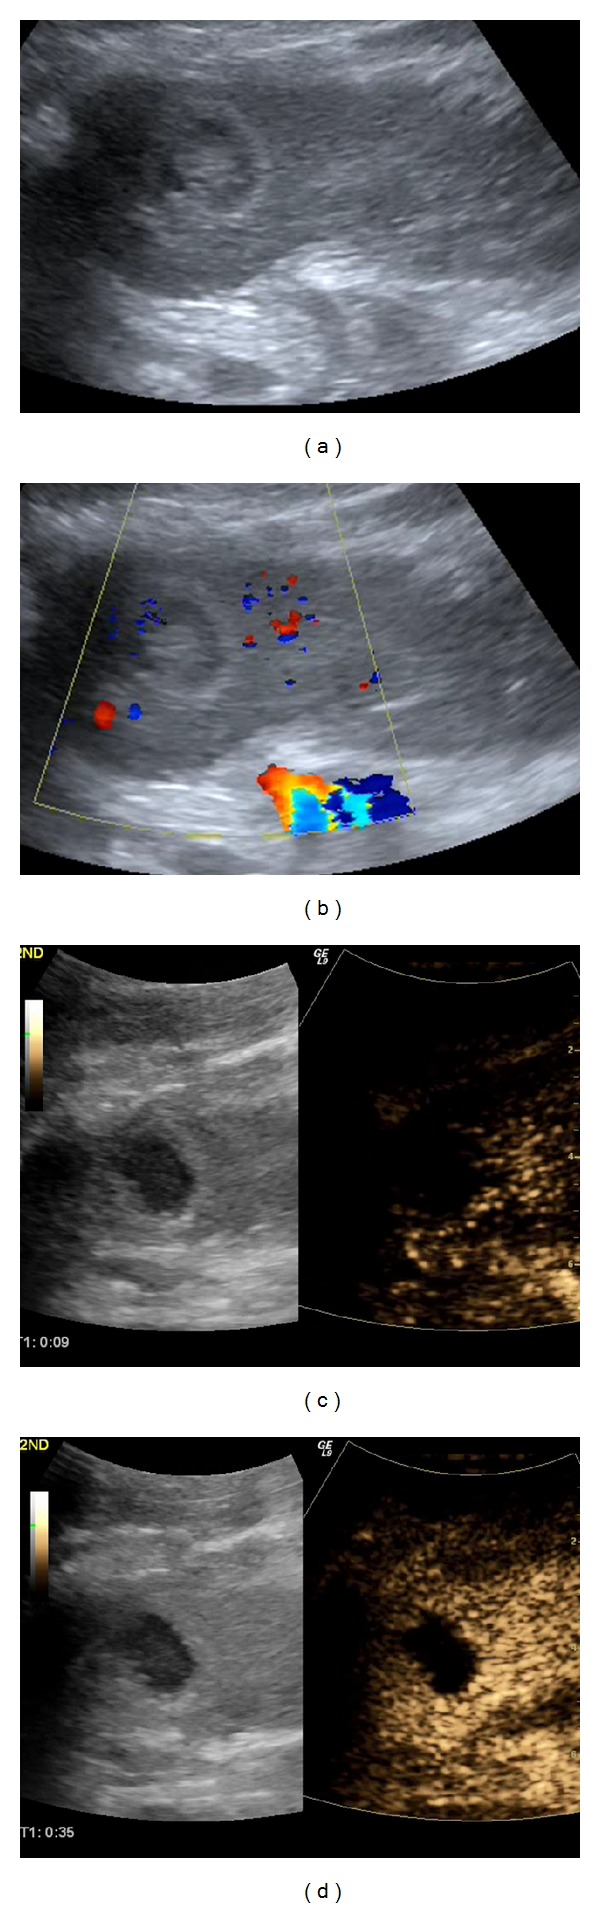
Renal abscess: B-mode (a) and Colour Doppler (b) US detect a round mixed echogenicity lesion in the upper part of the left kidney. After contrast injection, early enhancement is seen in the periphery of the lesion (c) with no internal uptake ((c), (d)).
